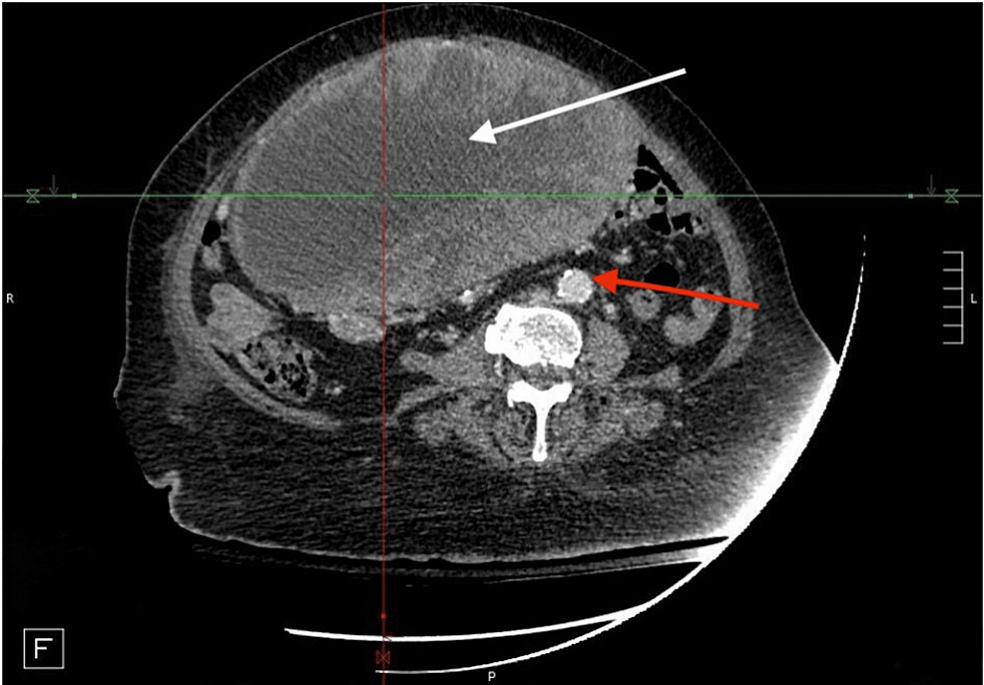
CT abdomen and pelvis CT Abdomen/pelvis horizontal/transverse plane re-demonstrating the large intraperitoneal mass (white arrow) marked with central necrosis and peripheral enhancement and lateral shift of the descending aorta from its medial baseline (red arrow)
